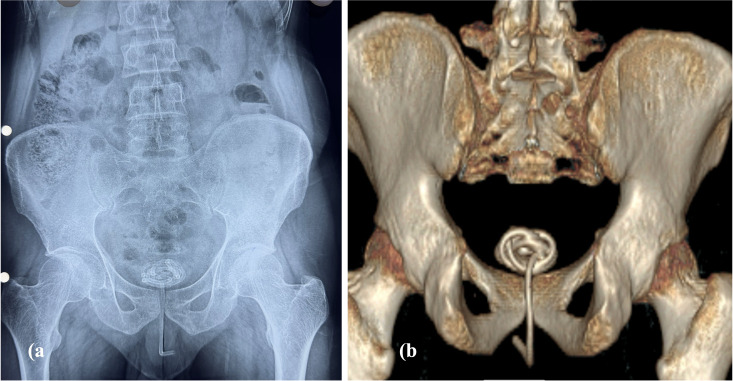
(A) X-ray KUB demonstrating a coiled wire superior to pubic symphysis, with one end extending into the urethra (B) 3D reconstructed image of CT scan showing coiled wire in bladder.
